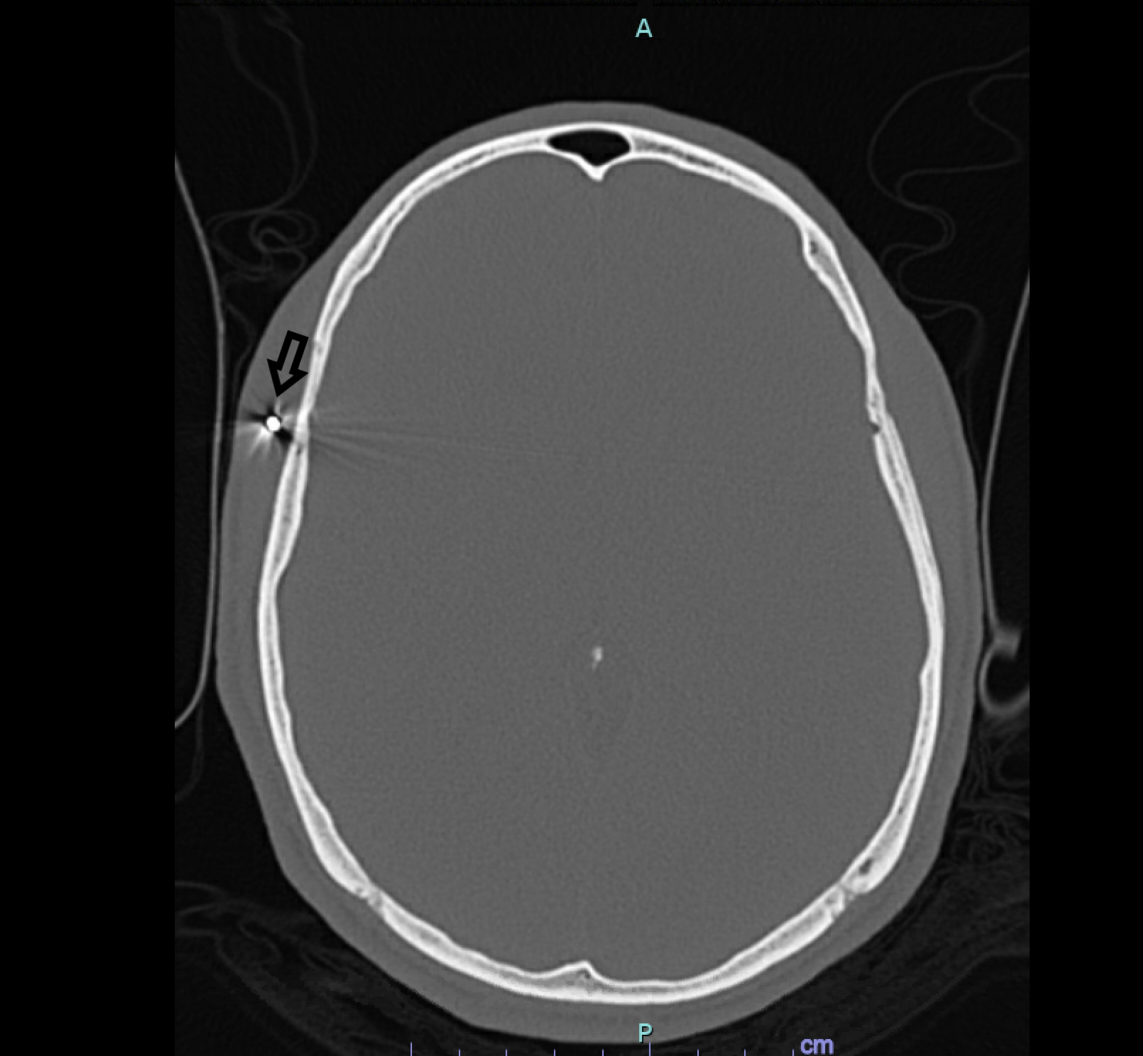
CT head without contrast Arrow=Pellet in right facial soft tissues
